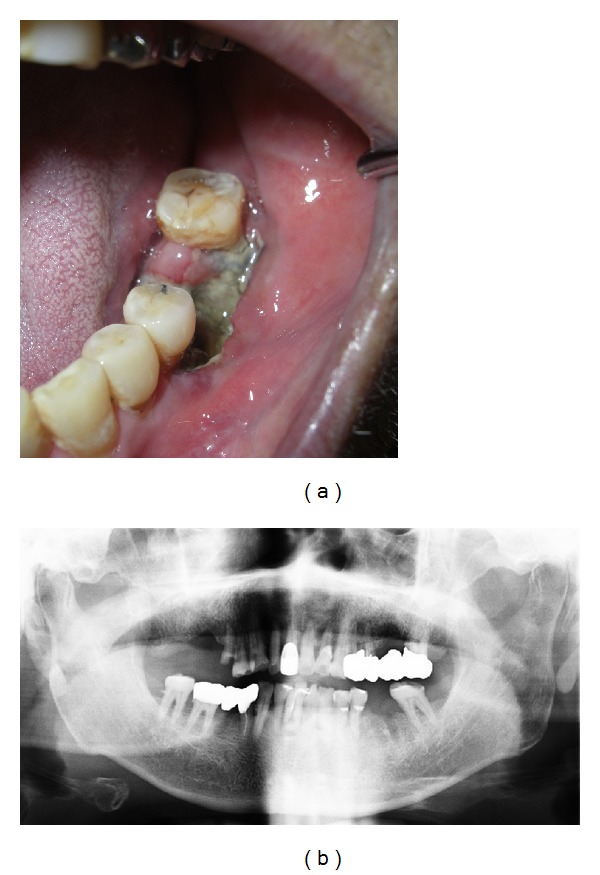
Clinical findings at the initial visit. (a) Close-up view of the socket in the left mandibular first molar region. (b) Panoramic radiograph showing neither abnormal consolidation nor ill-defined trabecular bone structure around the socket and clear running of the inferior alveolar arteries.
